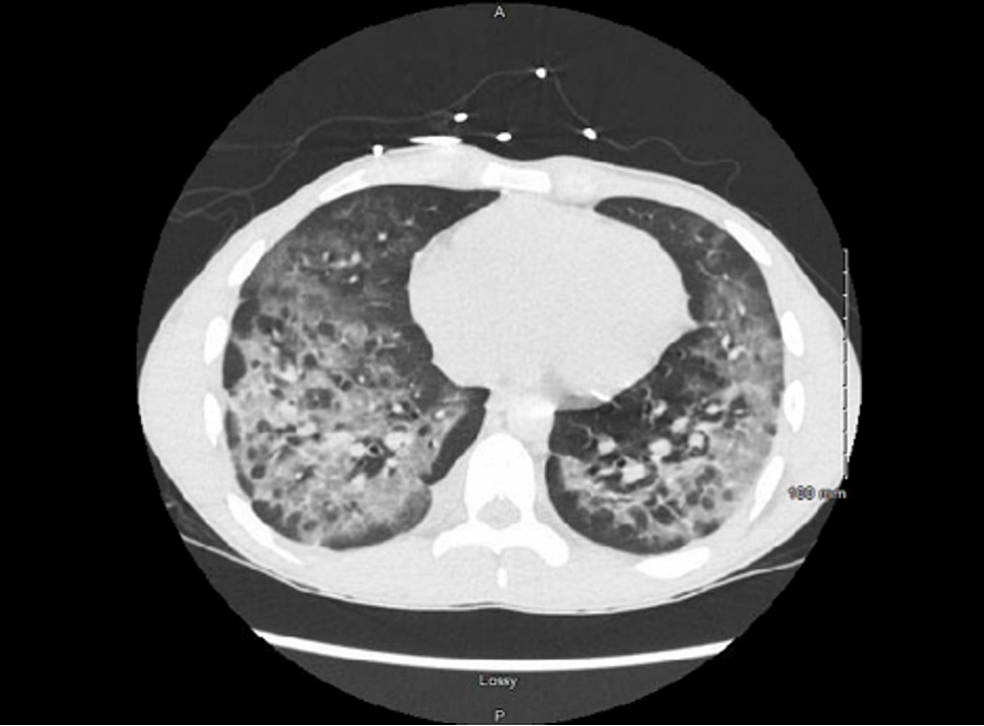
Chest CT at time of admission showing bilateral upper lobe ground-glass opacities.
